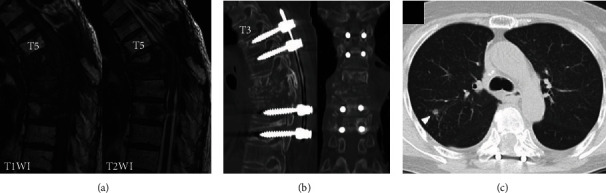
Imaging findings 9 months after surgery. (a) MRI sagittal imaging. (b) CT sagittal and coronal reconstruction. All the pedicle screws were loosened with the progression of the T6-7-8 vertebrae. (c) Chest CT. Arrowhead shows the coin lesion.
